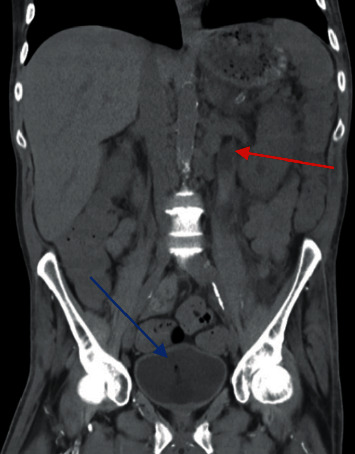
Coronal CT image showing evidence of ureteral fungal ball (red arrow) and emphysematous cystitis (blue arrow).
